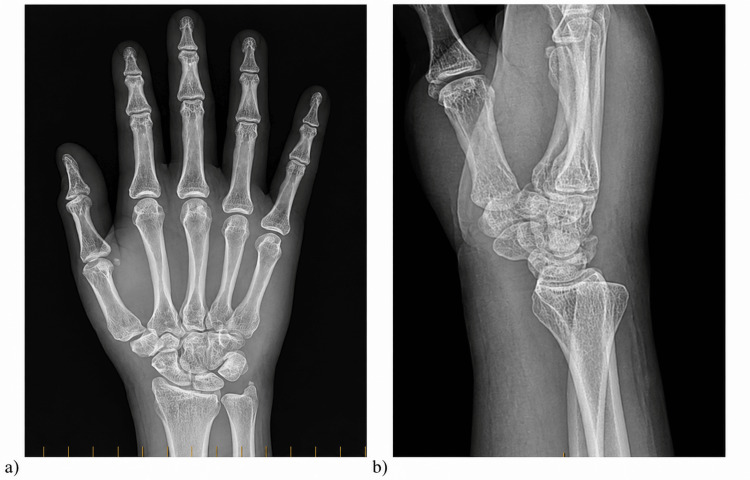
Plain radiographs obtained 10 days after the initial onset of symptoms without evidence of chondrolysis or joint destruction: a) anteroposterior view and b) lateral view.
